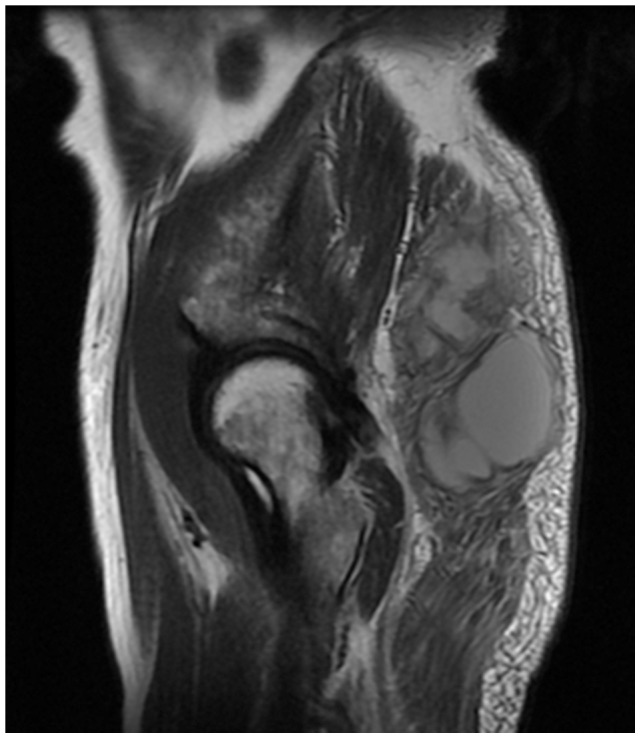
MRI scan of the hip on T1W1 reveals elevated signal intensity on the right gluteus maximus muscle. Massive irregular, homogeneous, mass-like abnormal signal intensity with unclear boundary was observed in the gluteus maximus muscle, measuring about 151 cm×90 cm, and subcutaneous edema-like signal intensities were observed in the right hip.
